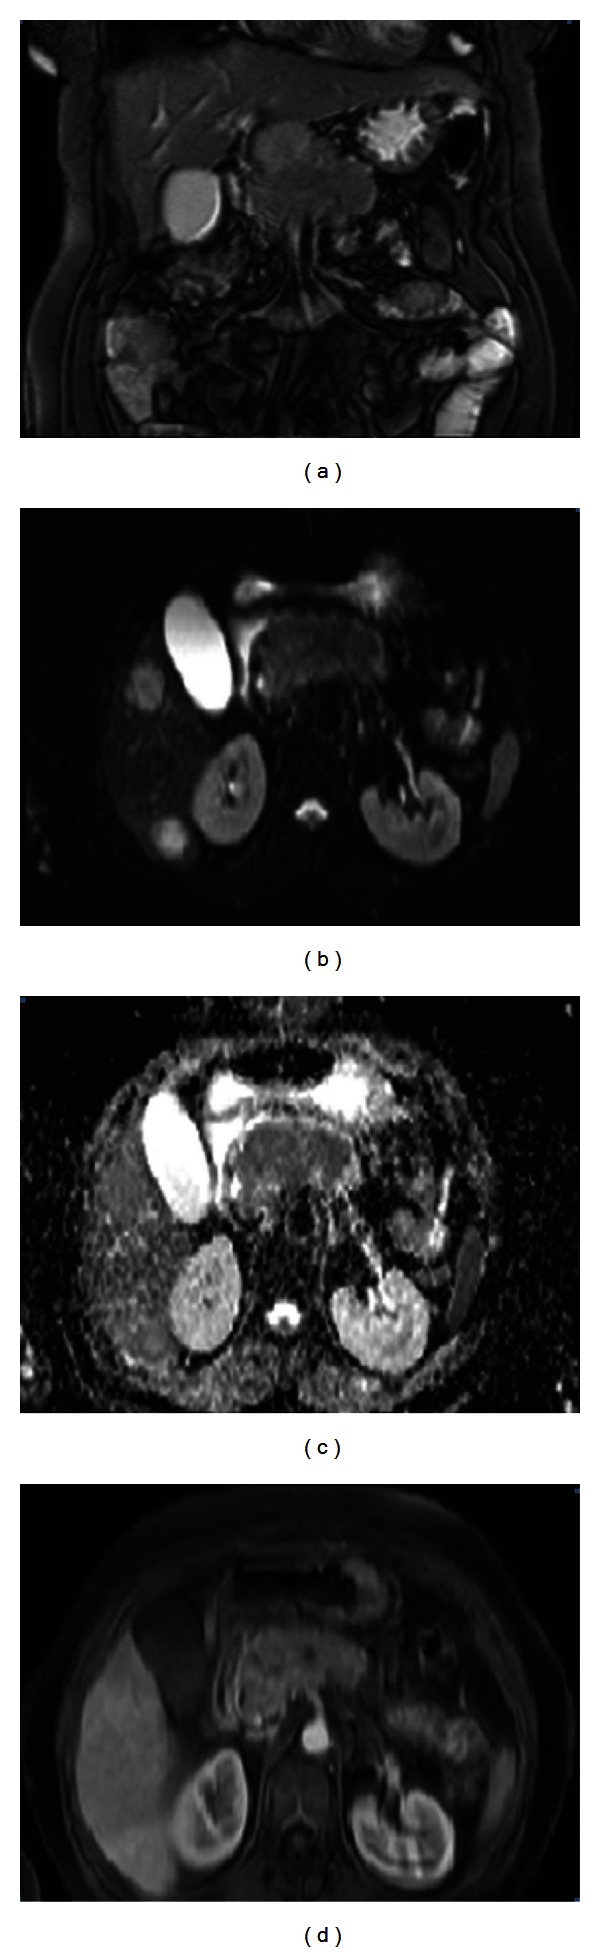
Coronal and axial images from initial abdomen MRI showing the primary tumour found between head and corpus of pancreas and invasion of the adjacent large vessels.
